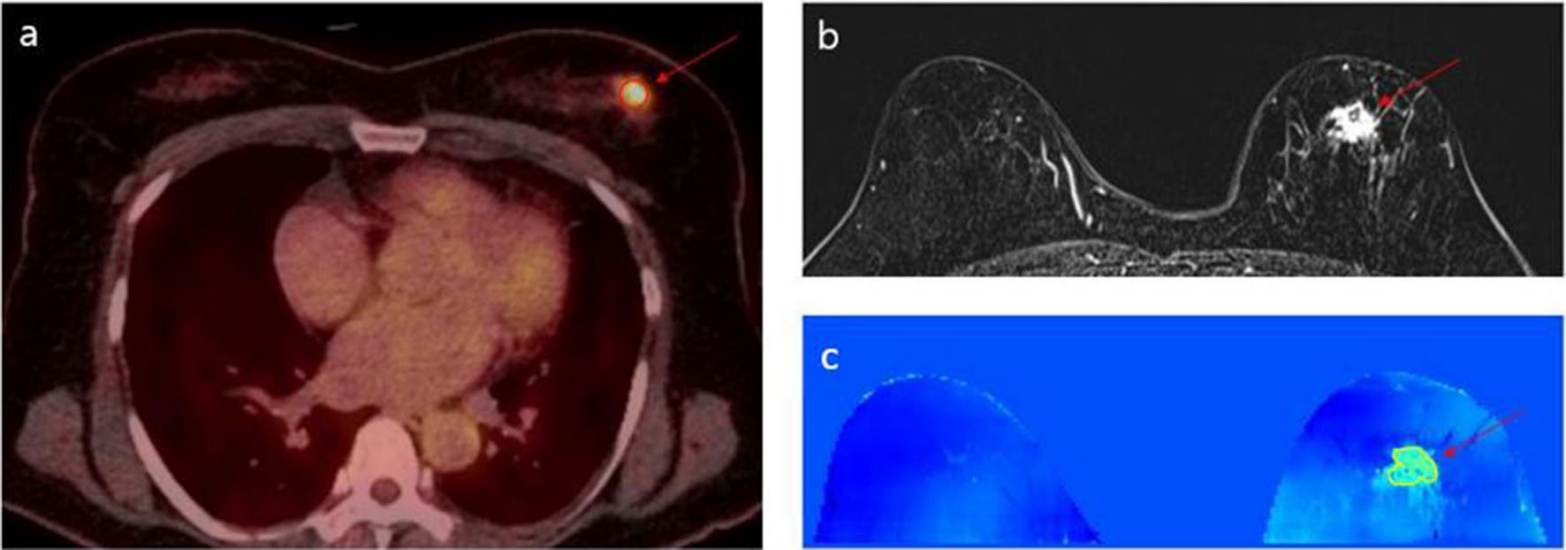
Images in a 55-year-old woman with a 3.2 cm human epidermal growth factor receptor type 2-positive invasive ductal carcinoma with lymphovascular invasion in left breast. (a) ^18^F-FDG PET/CT shows a high tumor SUVpeak value of 5.2 (arrow). (b) T1-weighted contrast-enhanced subtraction image shows an irregular heterogeneously enhancing mass (arrow). (c) Conductivity map shows a high tumor mean conductivity value of 0.33 S/m (arrow). Both SUV and conductivity measures showed high values in tumor with lymphovascular invasion.
